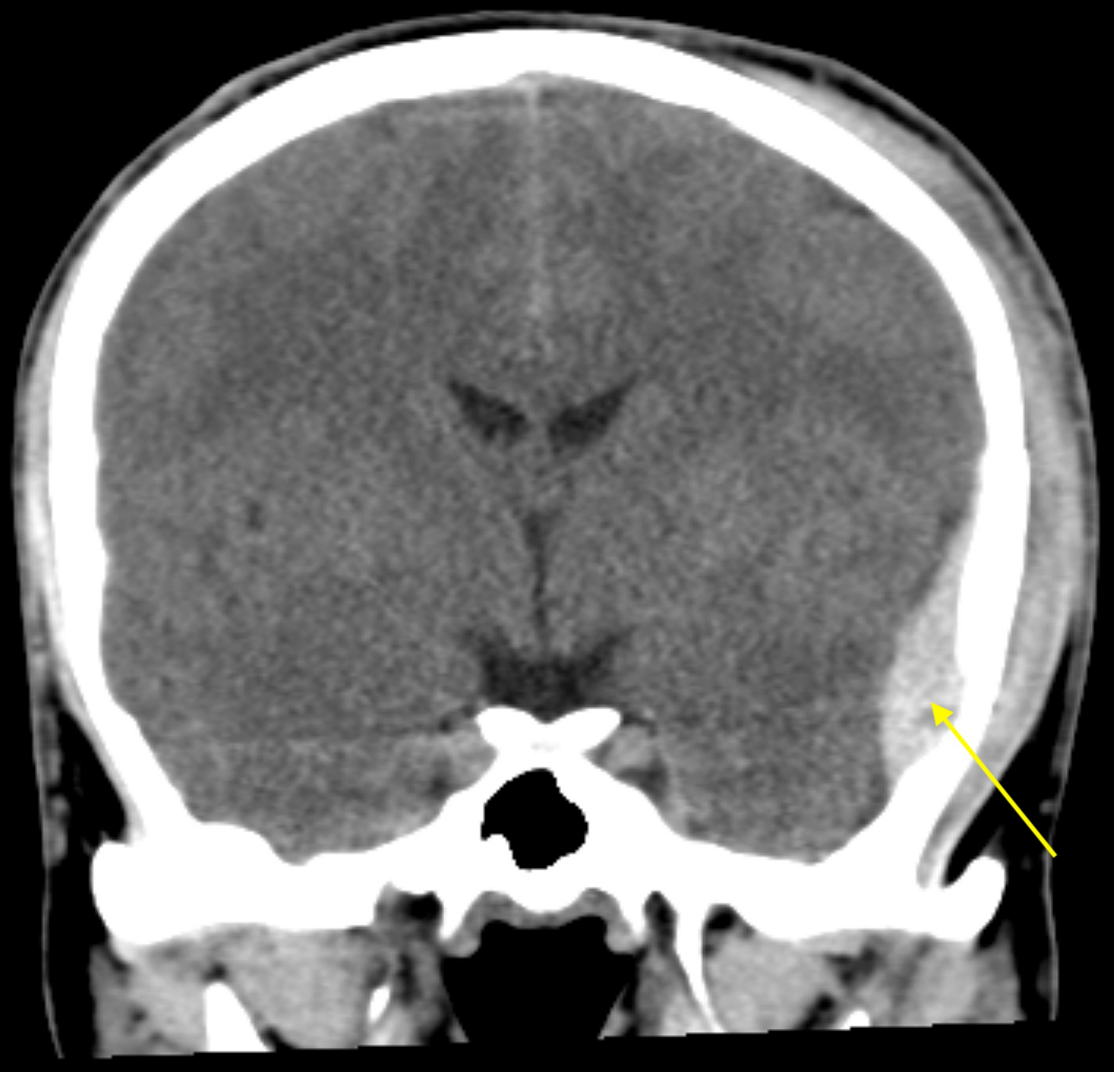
Non-contrasted coronal CT demonstrates hyperdense lentiform epidural hematoma in the left temporal lobe with maximum thickness measuring approximately 1.4 cm (yellow arrow).
